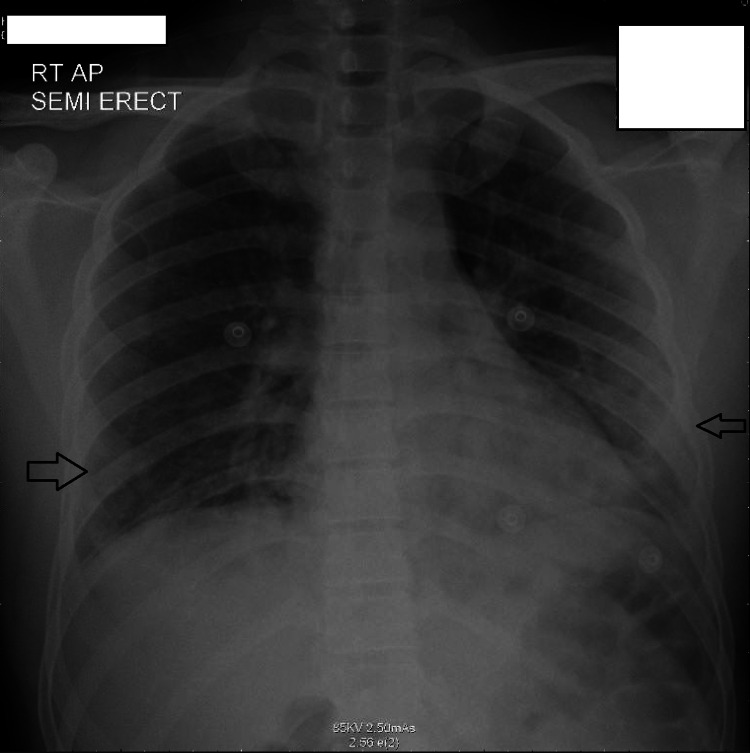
Chest X-ray showing multifocal airspace opacities noted in bilateral lower zone pattern of COVID-19 pneumonitis. COVID-19: Coronavirus disease 2019.
